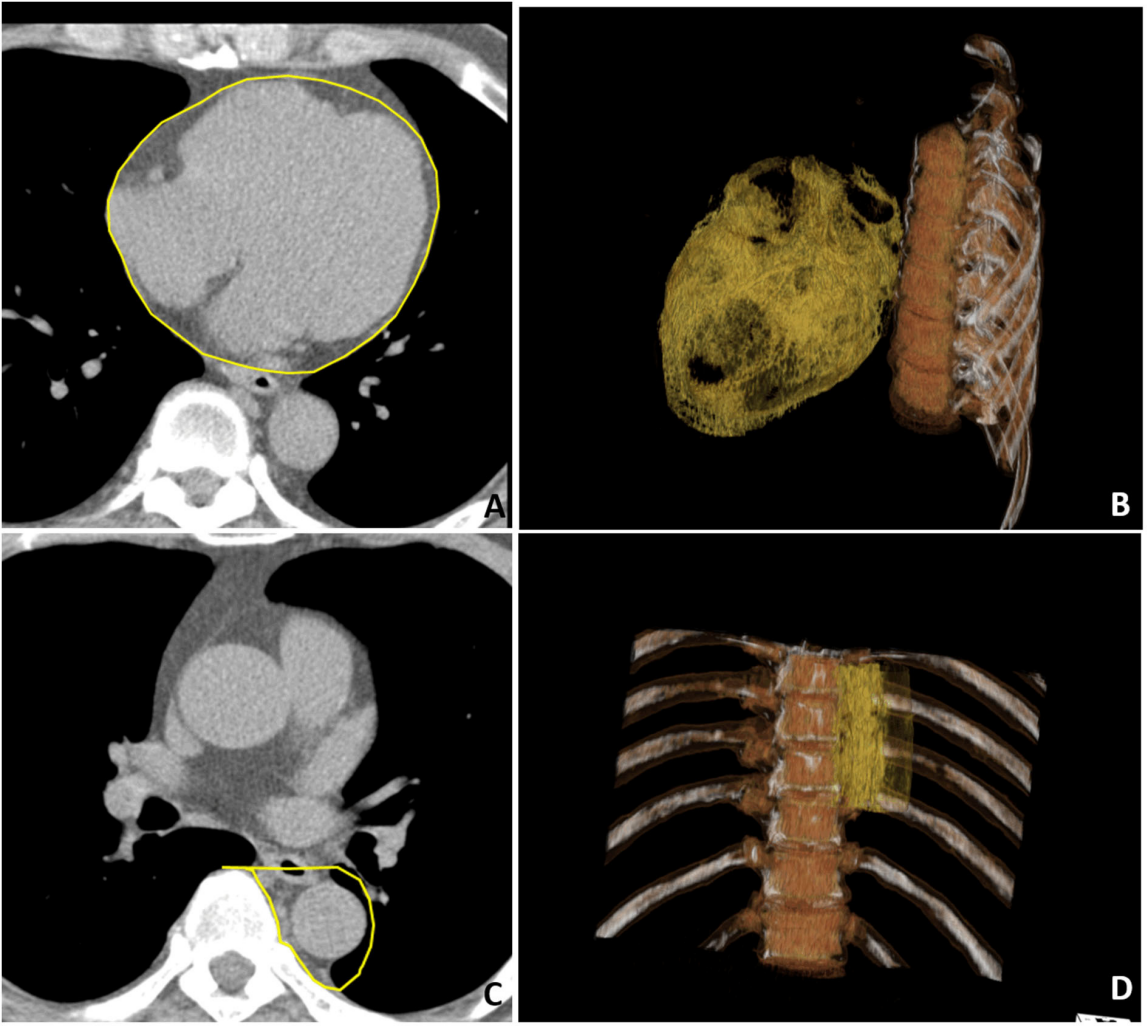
Multidetector computed tomography (MDCT) demonstrated regional-specific adiposity measures including (A,B) epicardial adipose tissue (EAT) and (C,D) peri-aortic fat (PAF) in (A,C) axial view and (B,D) three-dimensional (3D) reconstruction. (A) The EAT was defined as the fat inside the pericardial sac with pericardium labeled in yellow color. (C) The PAF was defined as the fat surrounding the thoracic descending aorta with the region of interest labeled in yellow. In the 3D reconstruction of the (B) EAT and (D) PAF, where orange regions indicate visceral fat tissue.
